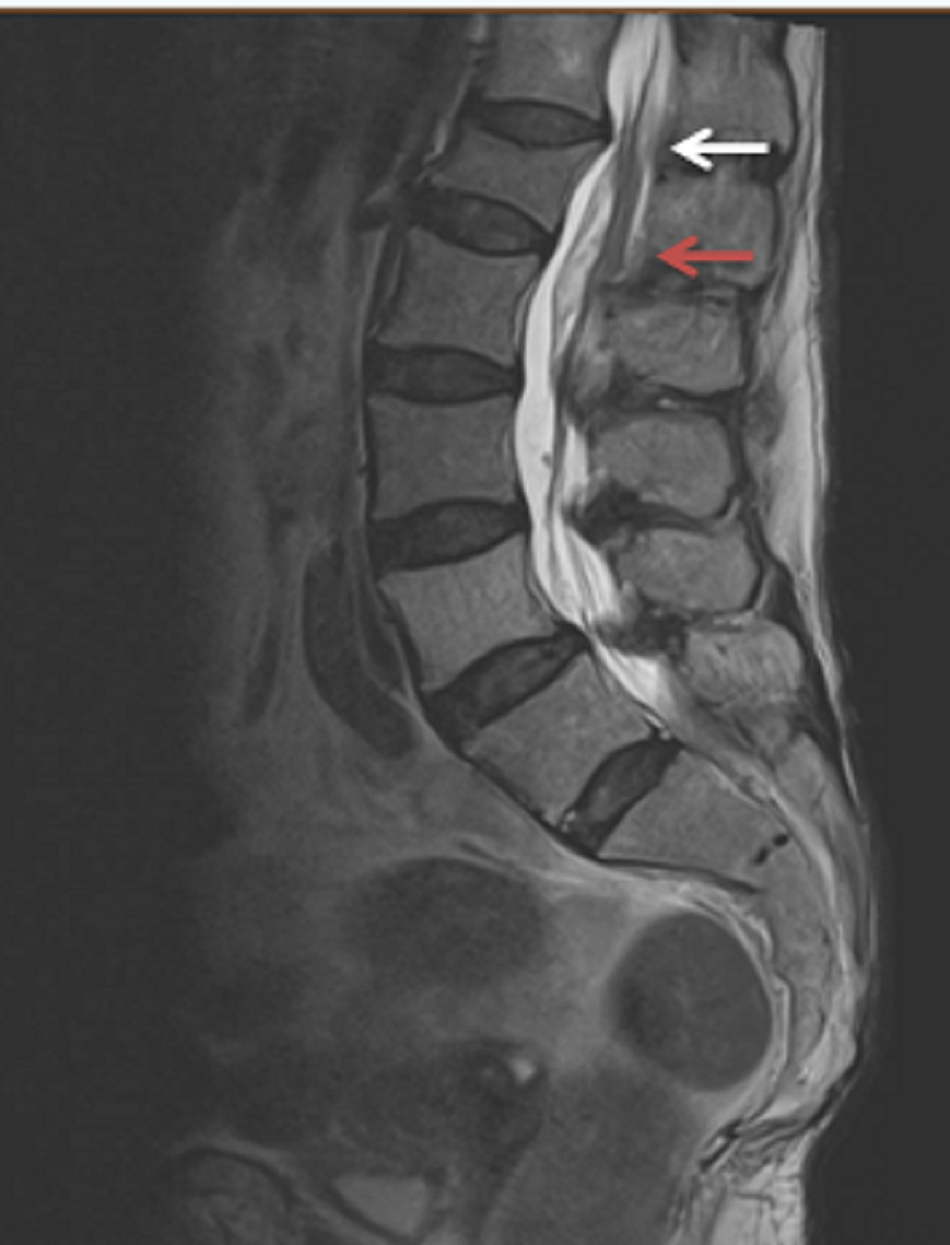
Sagittal T2-weighted sequence lumbar spine. The spinal subarachnoid haemorrhage is demonstrated as abnormal hypo to isointense signal intensity posterior to the cord at the thoracolumbar junction (white arrow) and lumbar region (red arrow) with associated narrowing.
